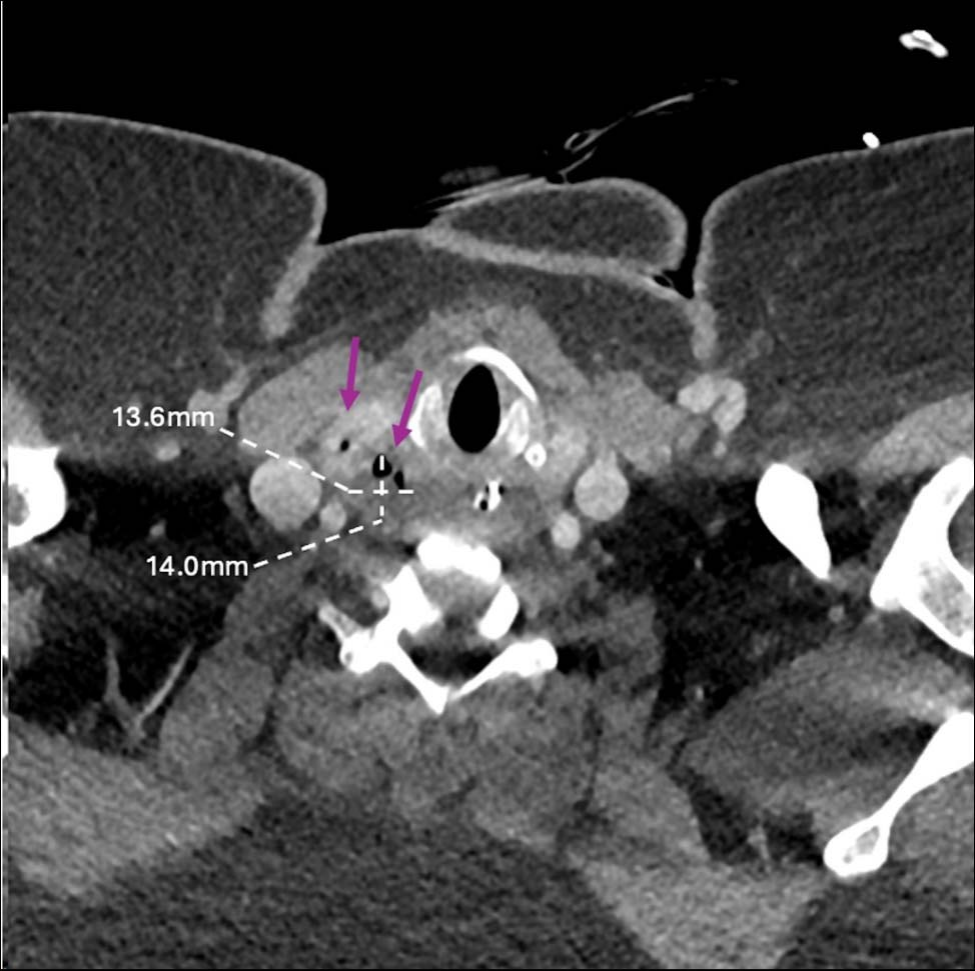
Computed tomography scan of neck with 1.36 cm × 1.4-cm sinus gas collection (purple arrows) between the right lateral margin of hypopharynx and superior thyroid.
